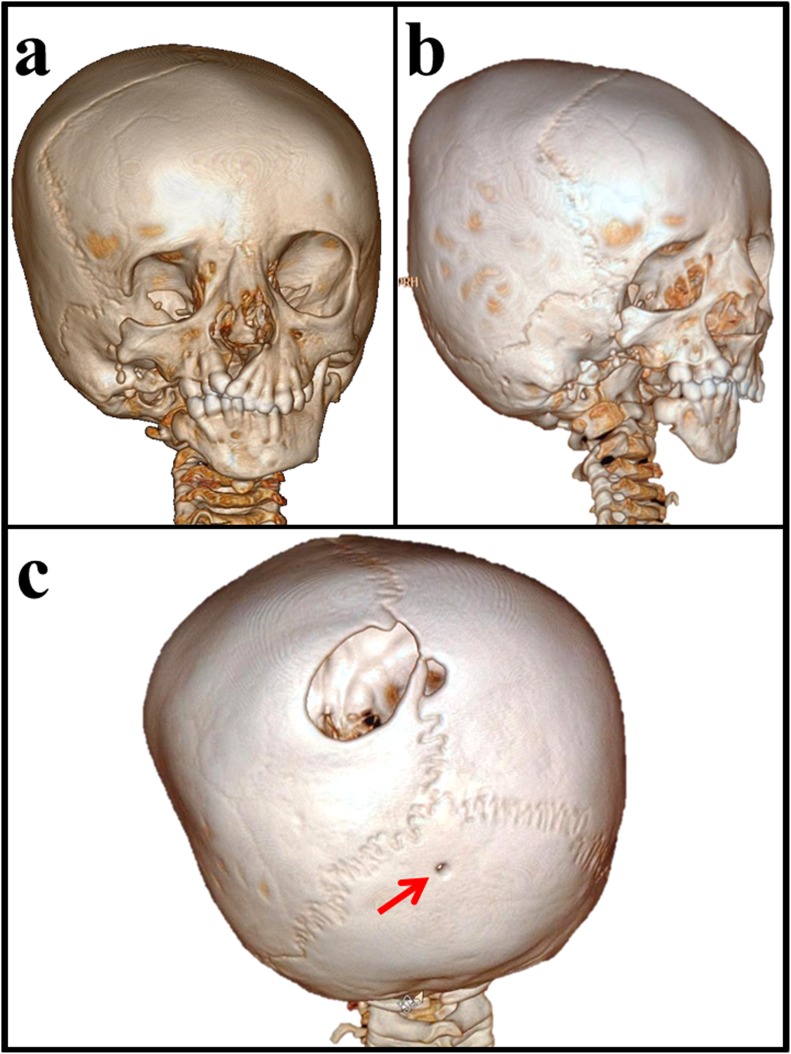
Three dimensional-CT scan of the skull a. Asymmetrical facial bones, small right orbit with a small sphenoid bone. Hypoplastic right zygomatic bone with missing of the frontal process, temporal process and the zygomatic arch. b. Hypoplastic right maxilla and temporal bone with missing of the right styloid process and cleft palate, in addition to missing of mandibular condyle, coronoid process, ramus and part of the body of the right mandible. c. Bilateral parietal osseous defects, represented asymmetrically enlarged parietal foramina, larger on the right side (3 × 2.5 cm) reaching the sagittal suture. A persistent occipital foramen at midline of occipital bone (*arrowed*).
